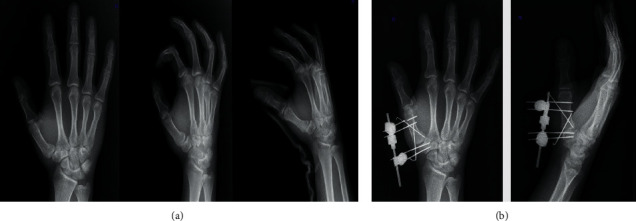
(a) Preop AP/lateral and oblique X-ray views of Bennet fracture. (b) Postop AP/lateral X-ray views of Bennet fracture.
